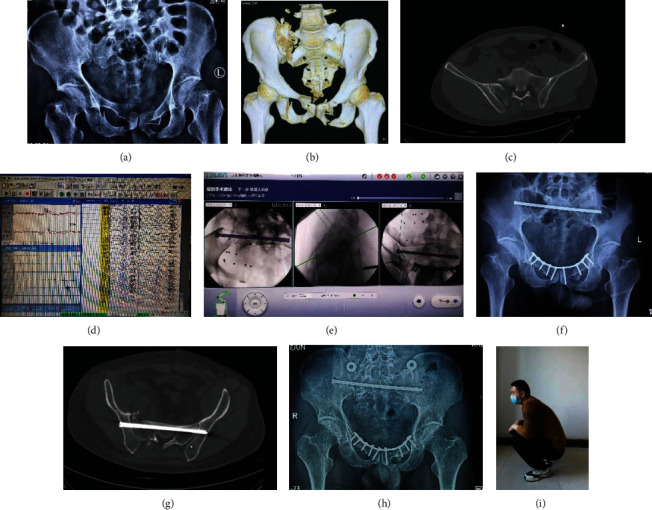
(a–i) A 49-year-old man presented with a pelvic fracture (AO/OTA C1.3) due to a fall. (a–c) Preoperative radiographs show bilateral pubic ramus fractures and transforaminal sacral fractures, and the right hemipelvis was vertically displaced. The patient presented with a dysmorphic sacrum. (d) During skeletal traction, the neuroelectrophysiological monitoring alert indicated that the SEP amplitude on the injured side (right) was lower than that on the contralateral side. After reducing the weight of traction, the SEP gradually recovered. (e) Robot-assisted planning for TITS screw fixation. (f, g) Postoperative radiographs showed that the TITS screw was in a good position. (h, i) The fracture healed, and the patient had good functional recovery 16 months postoperatively.
